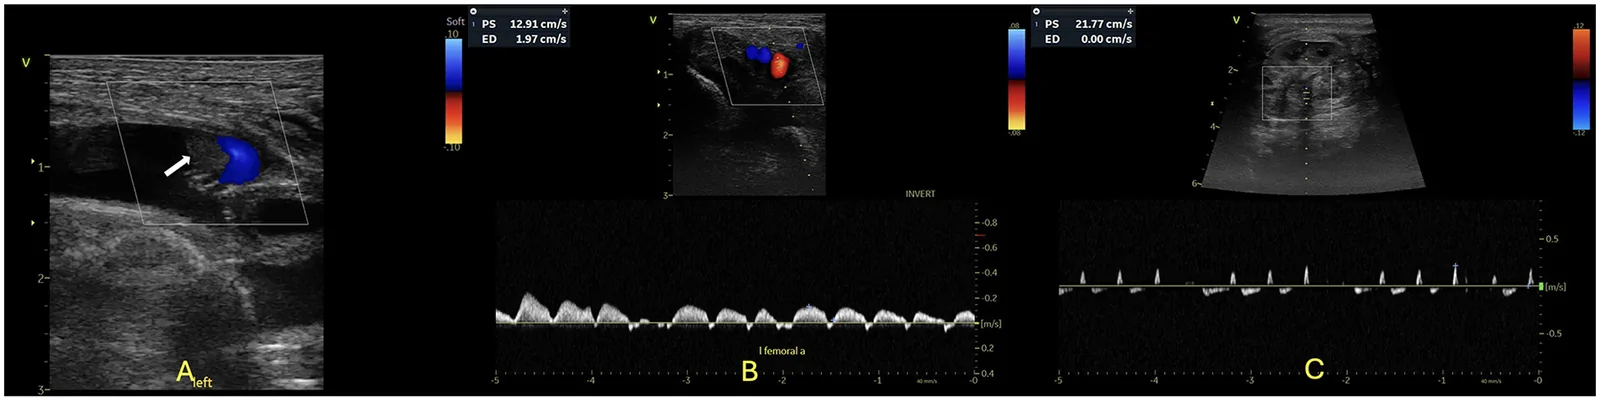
(A) Intravascular hypoechoic mass with residual flow within the left femoral artery, suggestive of a thrombus. (B) Spectral Doppler of the left femoral artery demonstrating markedly reduced peak systolic velocity. (C) Left renal artery Doppler with reduced peak systolic velocity and reversal of end-diastolic flow. (A,C) Reprinted with permission from Abdominal Aortic Thrombosis in a Preterm Baby With Congenital Diaphragmatic Hernia. Cureus 17(8): e89401. doi: 10.7759/cureus.89401 by: Goyal V, Jain N, Goyal M (August 05, 2025), licensed under under the Creative Commons Attribution License (CC BY 4.0).
